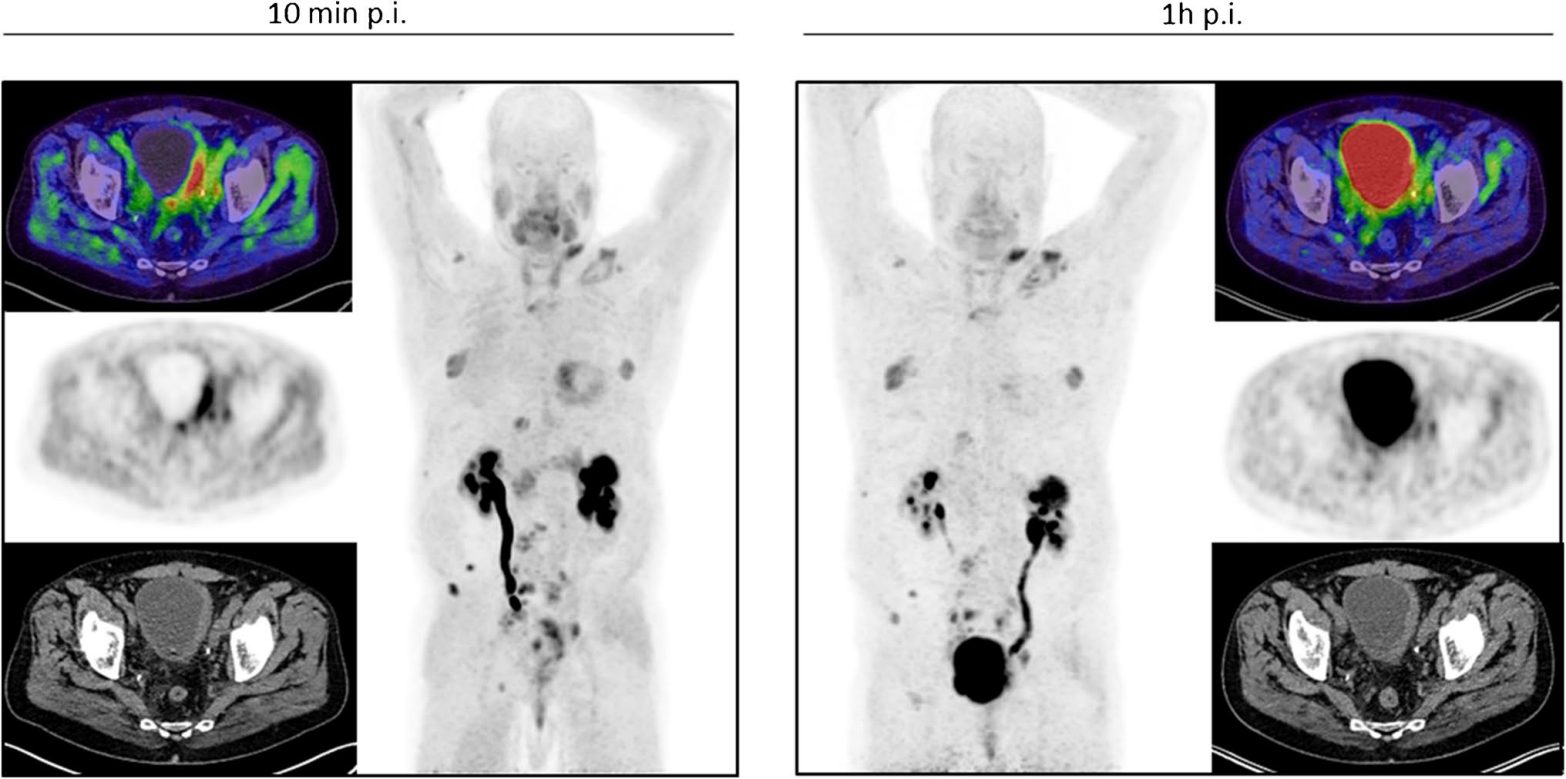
A 55-year-old male patient with a neuroendocrine metastasized urothelial bladder cancer showing similarly strong uptake 10 min (SUVmax of 9.32) and 1 h (SUVmax 9.39) after tracer administration
